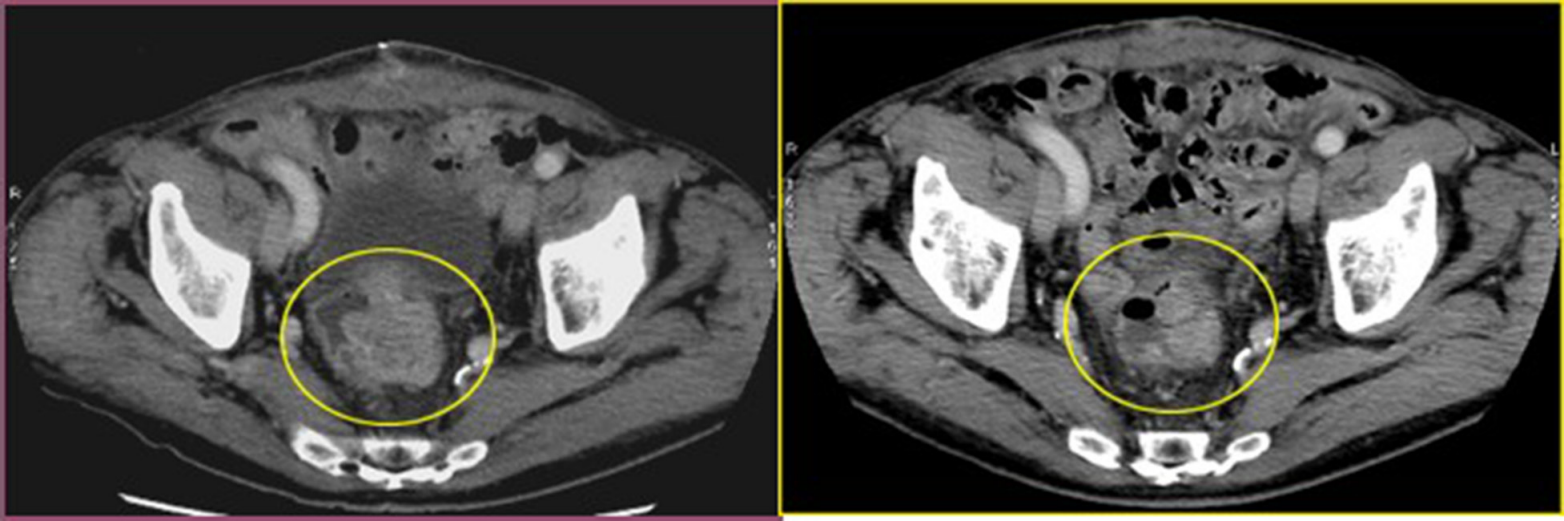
Cross section of contrast enhanced CT scan of the pelvis (venous phase) Left image: into the yellow circle tumor before chemoembolisation; Right image : into the yellow circle the reduction size of the lesion (30 day after treatment).
